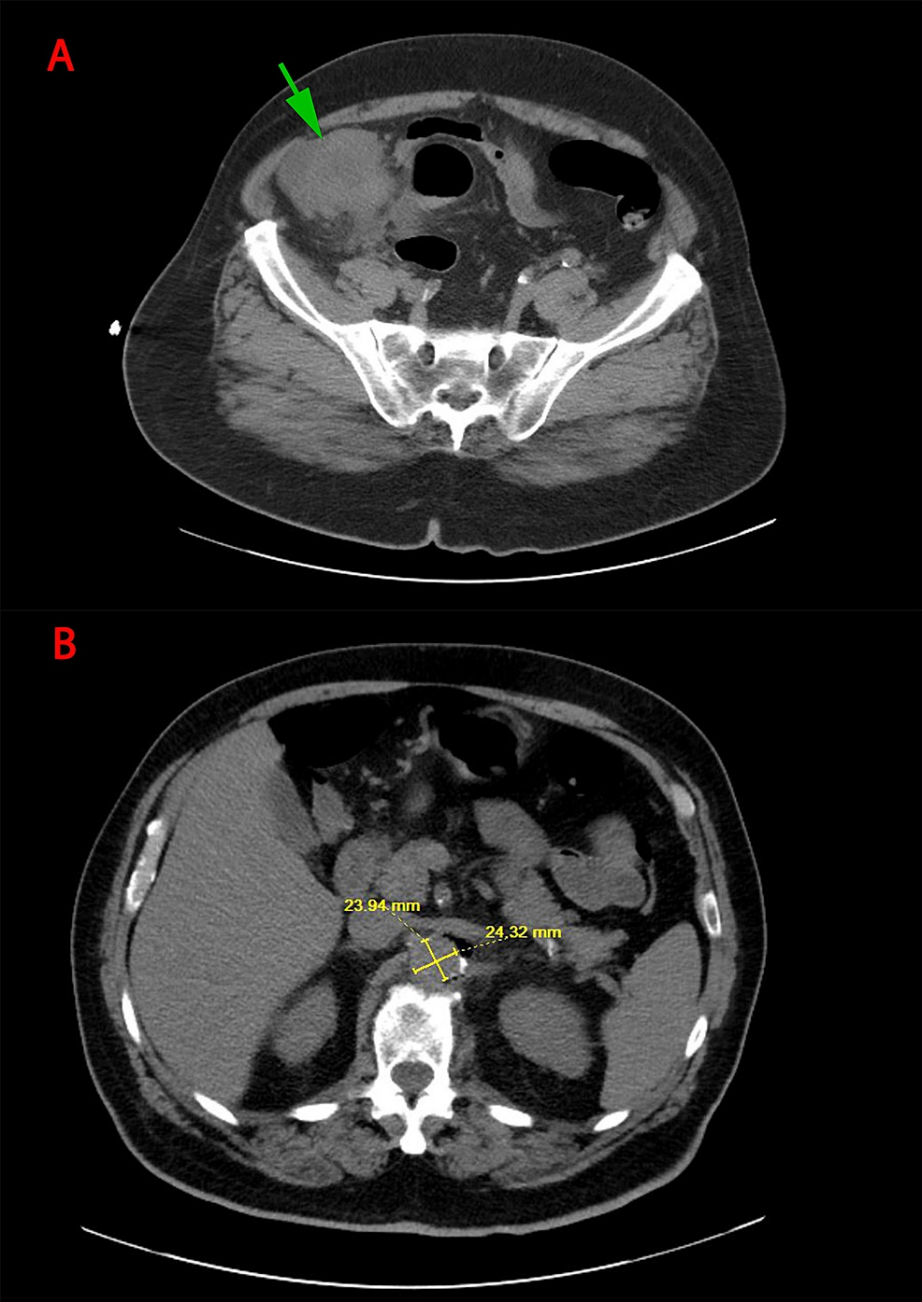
Computed tomography scan of the abdomen and pelvic at admission. A:  Mass in the cecum (green arrow) extending to the appendix and the ileocecal junction with severe adjacent inflammatory changes B:  Acute inflammatory changes were observed in the proximal abdominal aorta
